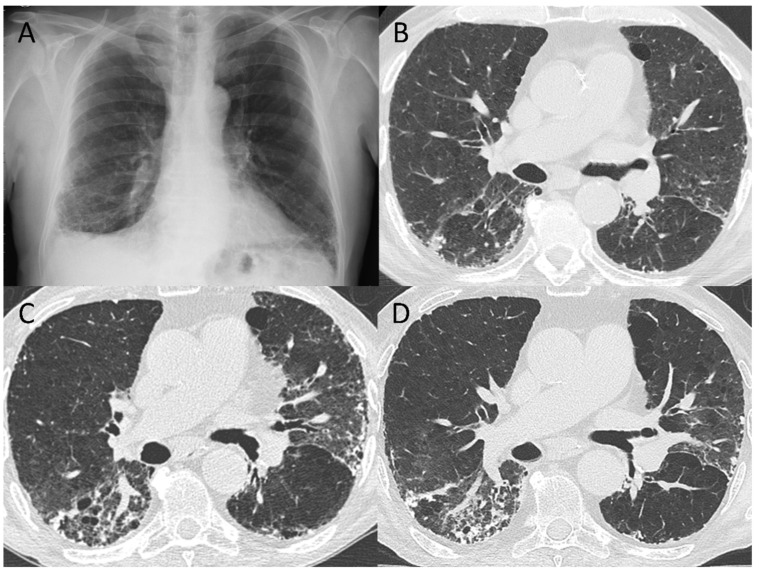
Representative images of a patient who had initial signs of interstitial lung disease at the lower lung zones in a chest X-ray (A) performed 2 years earlier than the baseline CT scan at COVID-19 diagnosis (B). In the 3-month (C) and 6-month (D) follow-up CTs the pattern was classified as residual fibrotic changes, possibly in keeping with COVID-19-induced progression of a pre-existing interstitial lung disease.
